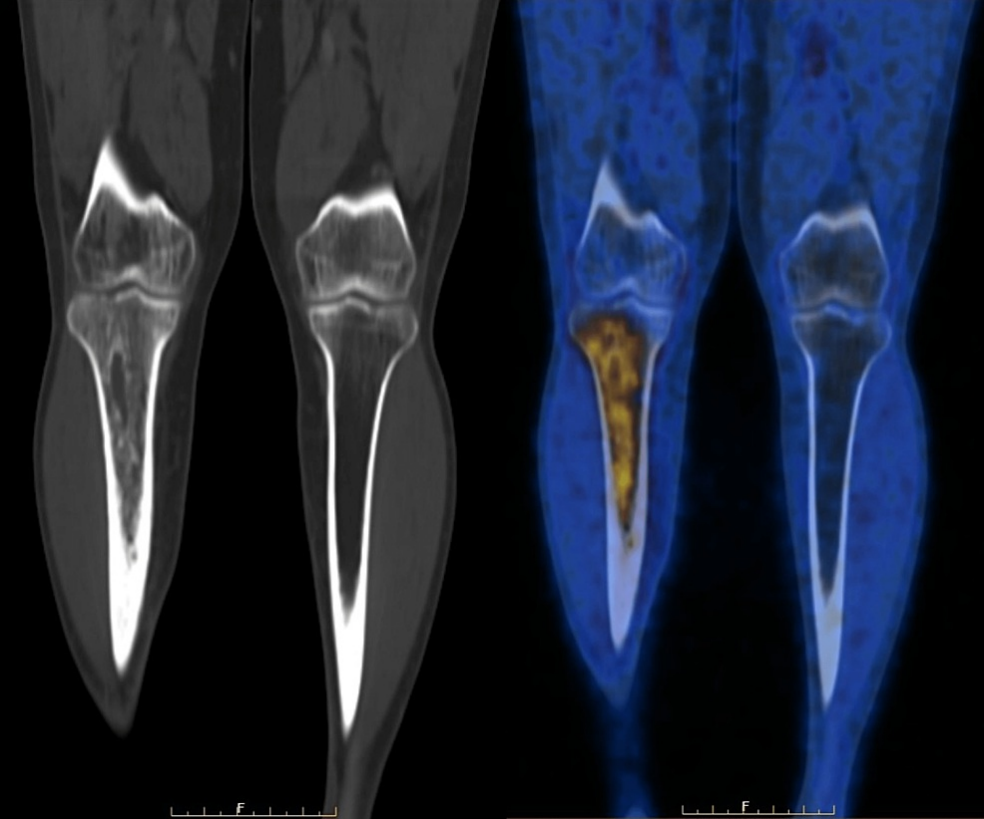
Positron emission tomography FDG avid permeative lytic lesions involving the diaphysis extending to the metaphysis and epiphysis of the right tibia - metabolically active proven primary lymphoma. FDG avid multiple right external iliac, right femoral and multiple lymph nodes above the popliteal fossa (largest measuring 13 mm × 6mm), suggestive of metabolically active lymphomatous deposits (not shown in the above figure) were also noted in this scan.
